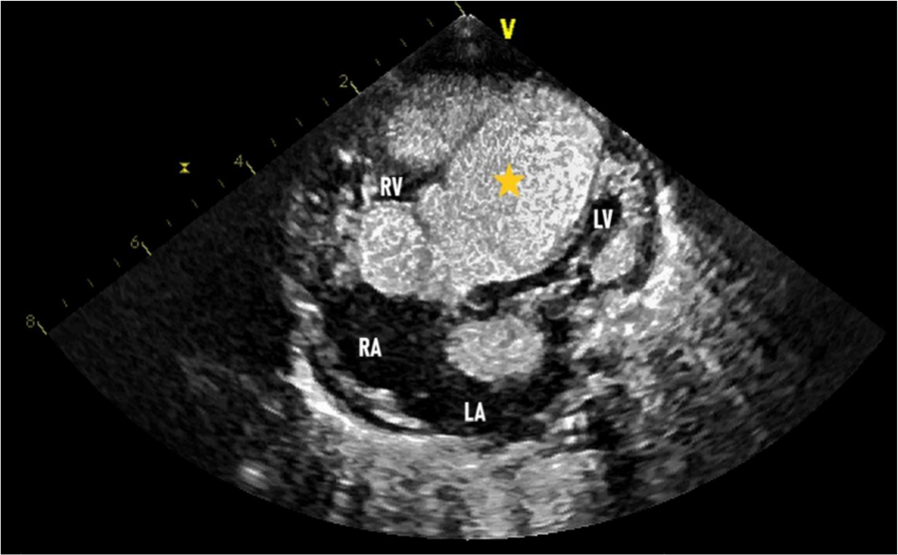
Apical Five Chamber View. Left Atrium (LA). Left ventricle (LV). Right Ventricle (RV). Right Atrium (RA). Asterisk showing the Hibernoma exerting mass effect on the LV
